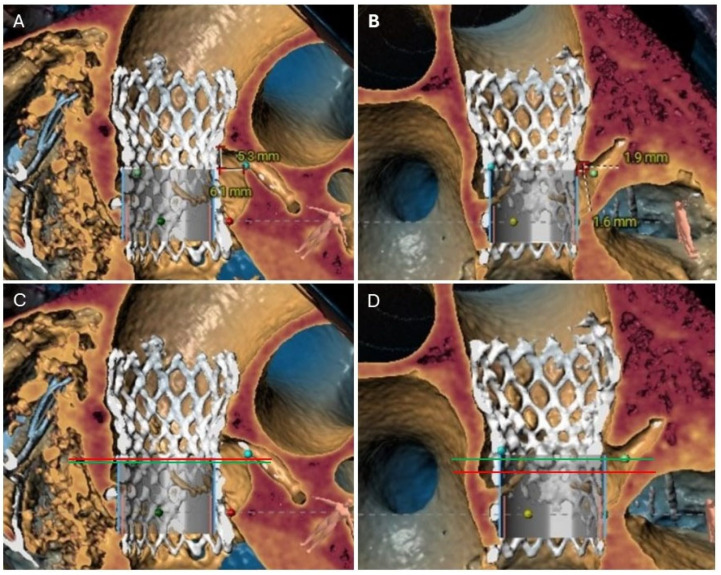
(A) 3D cardiac CTA demonstrating adequate VTC distance from the virtual S3 valve to LM. (B) On the contrary, the VTC distance to RCA is <2 mm, indicating a high risk for coronary occlusion if S3 is implanted at node 5 or above. (C) For LM, the NSP (green line) is at the level of CRP (red line), suggesting low risk for coronary occlusion. (D) However, for RCA, the NSP (green line) is above the CRP (red line), and coronary protection is recommended during redo-TAVR, especially with a low VTC on the right. CTA, computed tomography angiography; CRP, coronary risk plane; NSP, Neoskirt plane; LM, left main; RCA, right coronary artery; VTC, valve to coronary.
